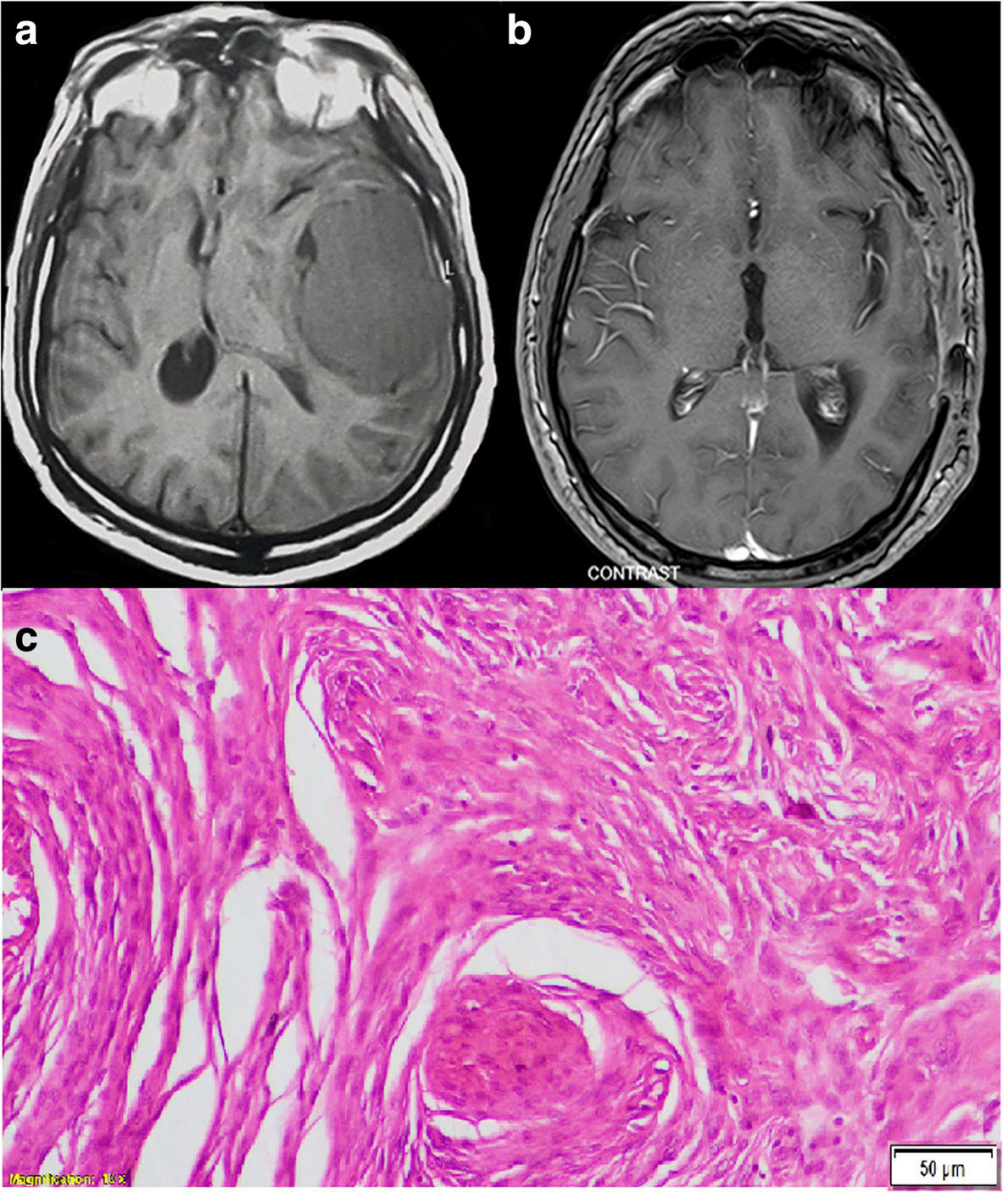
A convexity meningioma. a Preoperative image, MRI without contrast (axial) verified mass lesion in the right temporal region. The tumor was surrounded by mild edema. b Two year postoperative MRI with contrast (axial) demonstrated complete removal of the mass. Geriatric Scoring System (GSS) score = 16. c The slide represented transitional meningioma, WHO grade I
